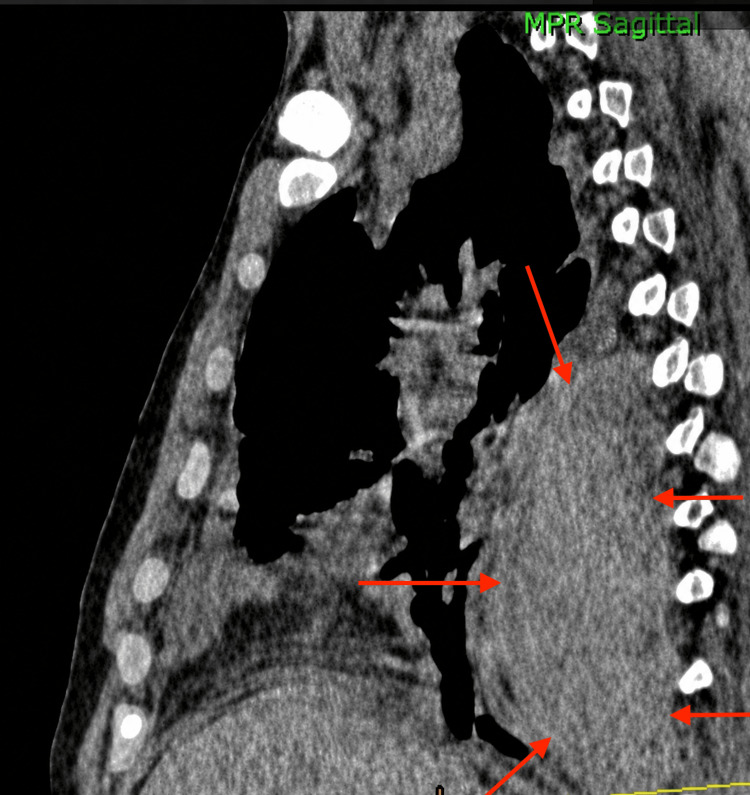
Sagittal non-contrast CT reconstruction showing extrapleural localization of the hematoma with compression of adjacent lung parenchyma.
